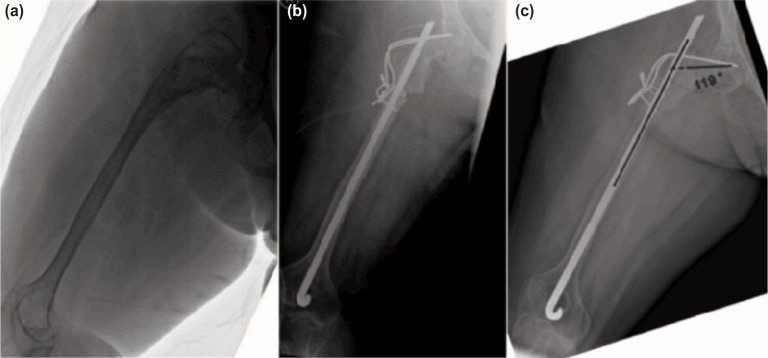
A series of radiographs of the 14-year old girl with osteogenesis imperfecta: (a) The preoperative radiograph shows NSA of less tha 90 degree and external malrotation (b) Immediate post-operative radiograph in Oct, 2013 with subtrochanteric osteotomy to increase NSA and correction of external rotation (c) Radiograph at one year after correction with union, maintenance of corrected NSA and rotation.
